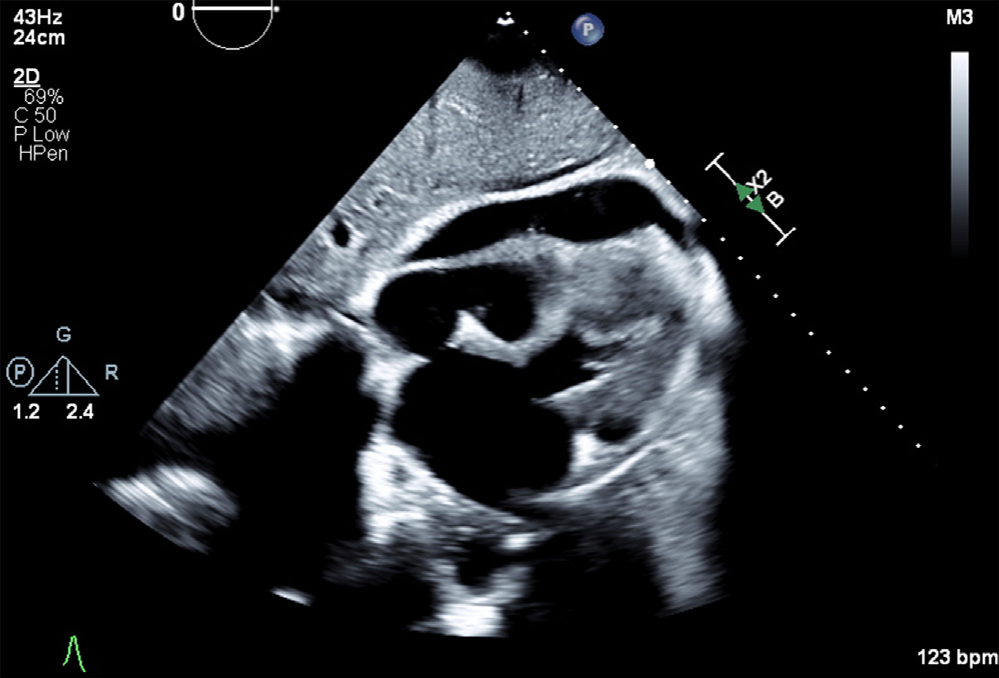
Transthoracic Echocardiogram Subcostal view with new-onset moderate, predominantly anterior, pericardial effusion.
